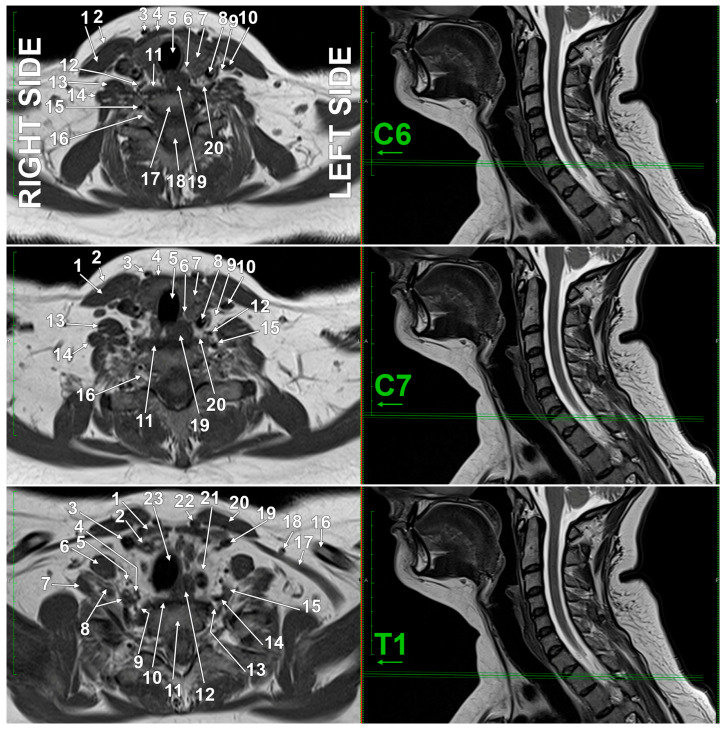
Sequence of original axial T2-weighted MRI slices through the C6, C7, and T1 vertebrae. Inferior views. The vascular topography on axial slices shows bilateral asymmetry. The scanning parameters were detailed in the previous legend. The C6 and C7 slices are similar; in these slices were identified the sternocleidomastoid muscle (1), platysma muscle (2); anterior jugular vein (3); strap muscles (4); trachea (5); recurrent laryngeal nerve (6); thyroid lobe (7); common carotid artery (8); vagus nerve (9); internal jugular vein (10); longus colli muscle (11); inferior thyroid artery (12); anterior scalene muscle (13); middle scalene muscle (14); vertebral artery (15); spinal nerve (16); vertebral body (17); spinal cord (18); esophagus (19); and the sympathetic trunk (20). At T1 there are identified the strap muscles (1); right common carotid artery (2); right internal jugular vein (3); right vertebral artery (4); right thyrocervical trunk (5); right anterior scalene muscle (6); right middle scalene muscle (7); right subclavian artery (8); right sympathetic trunk/stellate ganglion (9); longus colli muscle (10); vetebral body (11); esophagus (12); left sympathetic trunk/stellate ganglion (13); left vertebral artery (14); left subclavian artery (15); clavicle (16); omohyoid muscle (17); external jugular vein (18); left internal jugular vein (19); strap muscles (sternohyoid, sternothyroid) (20); left common carotid artery (21); anterior jugular vein (22); trachea (23).
